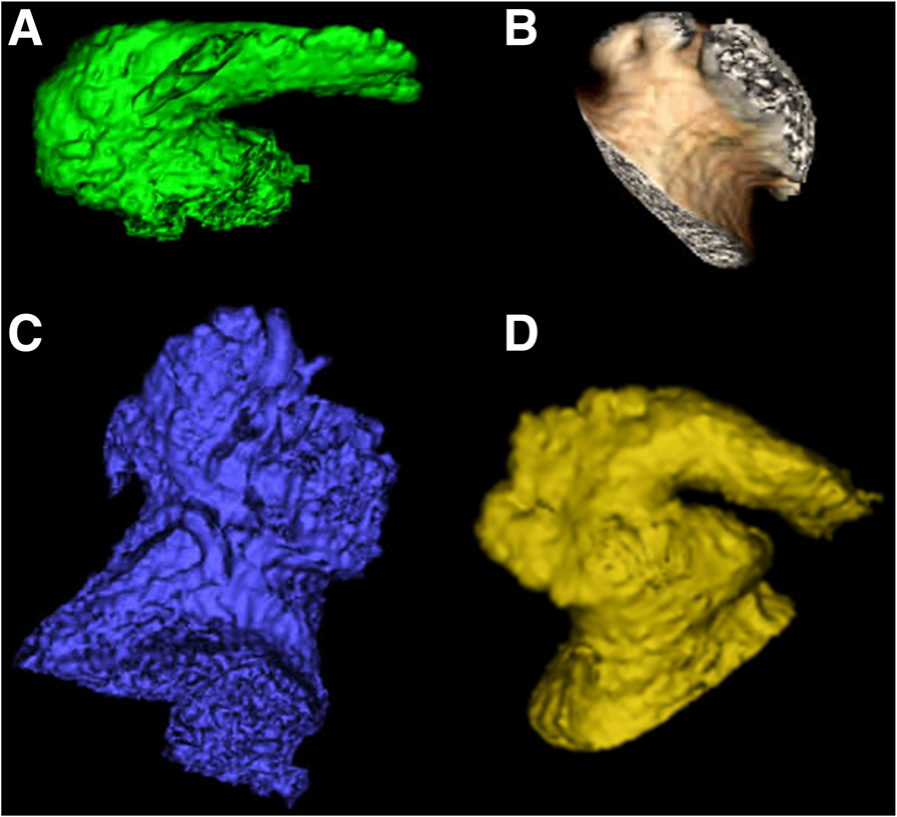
Volume rendered cardiac CT angiography images demonstrating chicken wing (a), cauliflower (b), cactus (c), and windsock (d) morphologies
